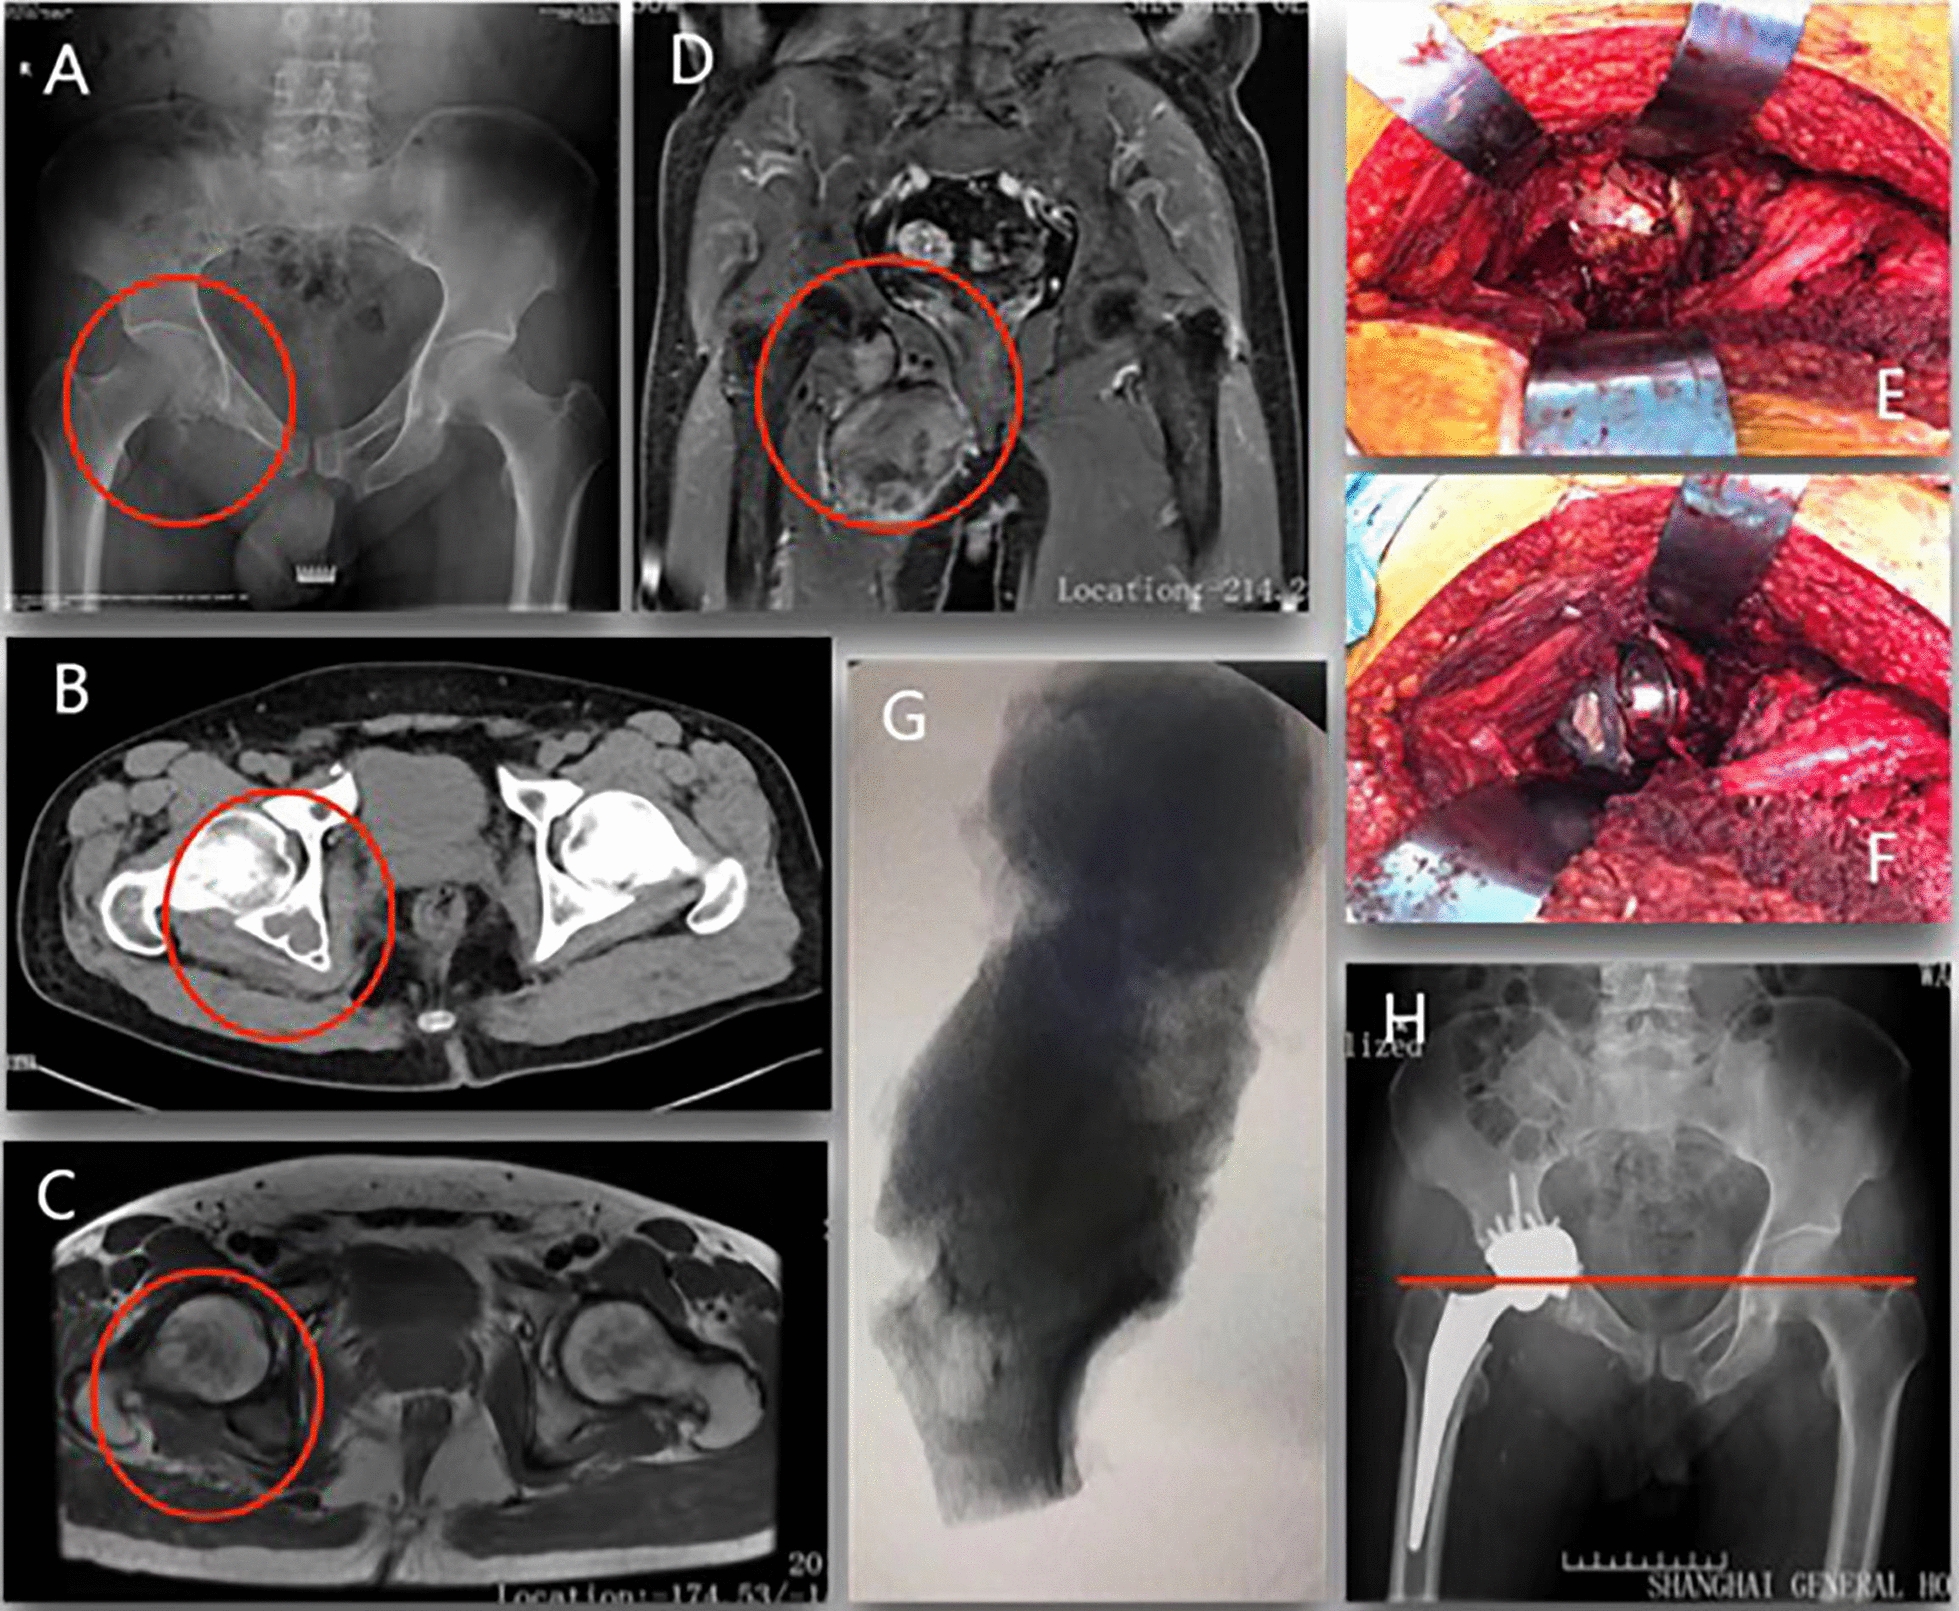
A male patient, diagnosed with pelvic MBGCT (Enneking Zone II + III, type2 defects). A: preoperative X-ray indicates tumor located at the right side of acetabulum; B: preoperative CT scan indicates the tumor involved the lower part of the acetabular; C, D: preoperative MR images indicate huge soft tissue mass involved acetabulum and ischium; E: intraoperative image showed the tumor was completely removed with dome of the acetabular reserved; F: intraoperative image showed the acetabular was reconstructed with tantalum component and bone cement; G: X-ray image of the resected tumor; H: pelvic X-ray at 1-year postoperatively indicates a stable internal fixation and alignment
